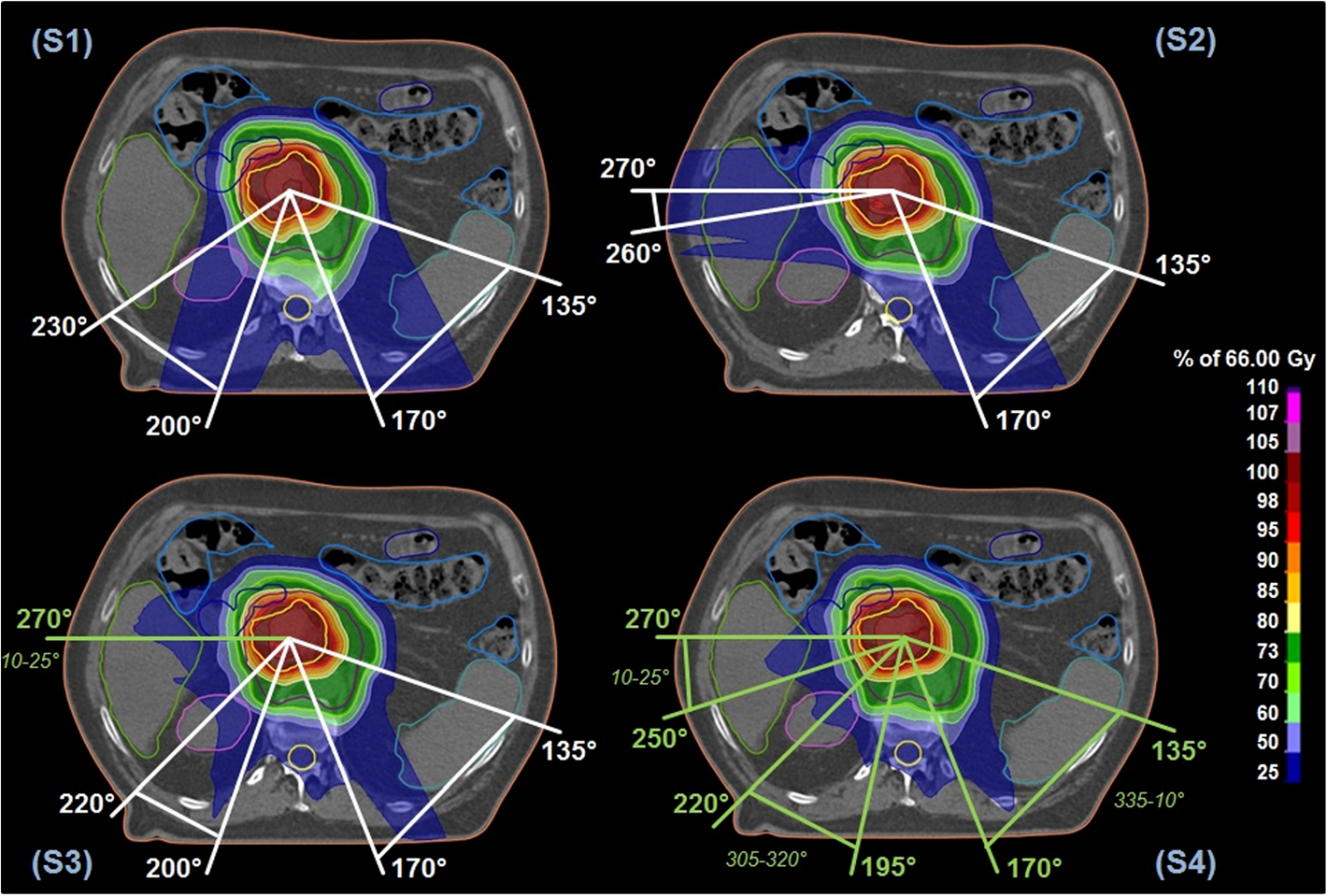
Overview of the beam configurations and resulting dose distribution of the four different treatment planning strategies (S1-S4) evaluated in this in silico treatment planning study. Coplanar beam directions are marked in white, non-coplanar beam directions in green. Moreover, the range of applied beam and couch angles in the six patients is given per beam direction. For patient 2, the resulting dose distribution to the CTV (violet) and GTV (orange) is shown as color wash superimposed on the planning CT
